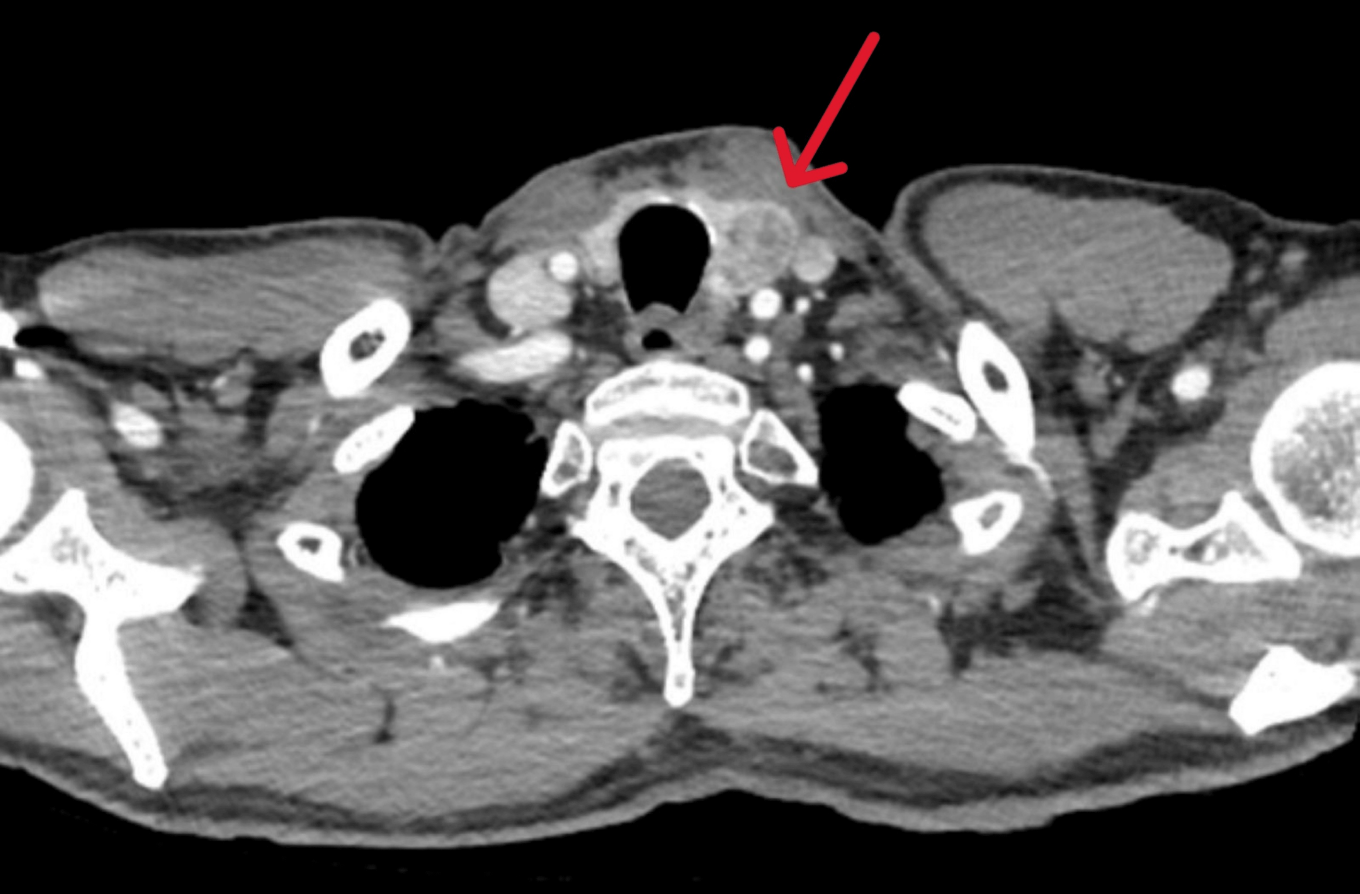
Axial CT image showing a left thyroid nodule (arrow). CT, Computed tomography
